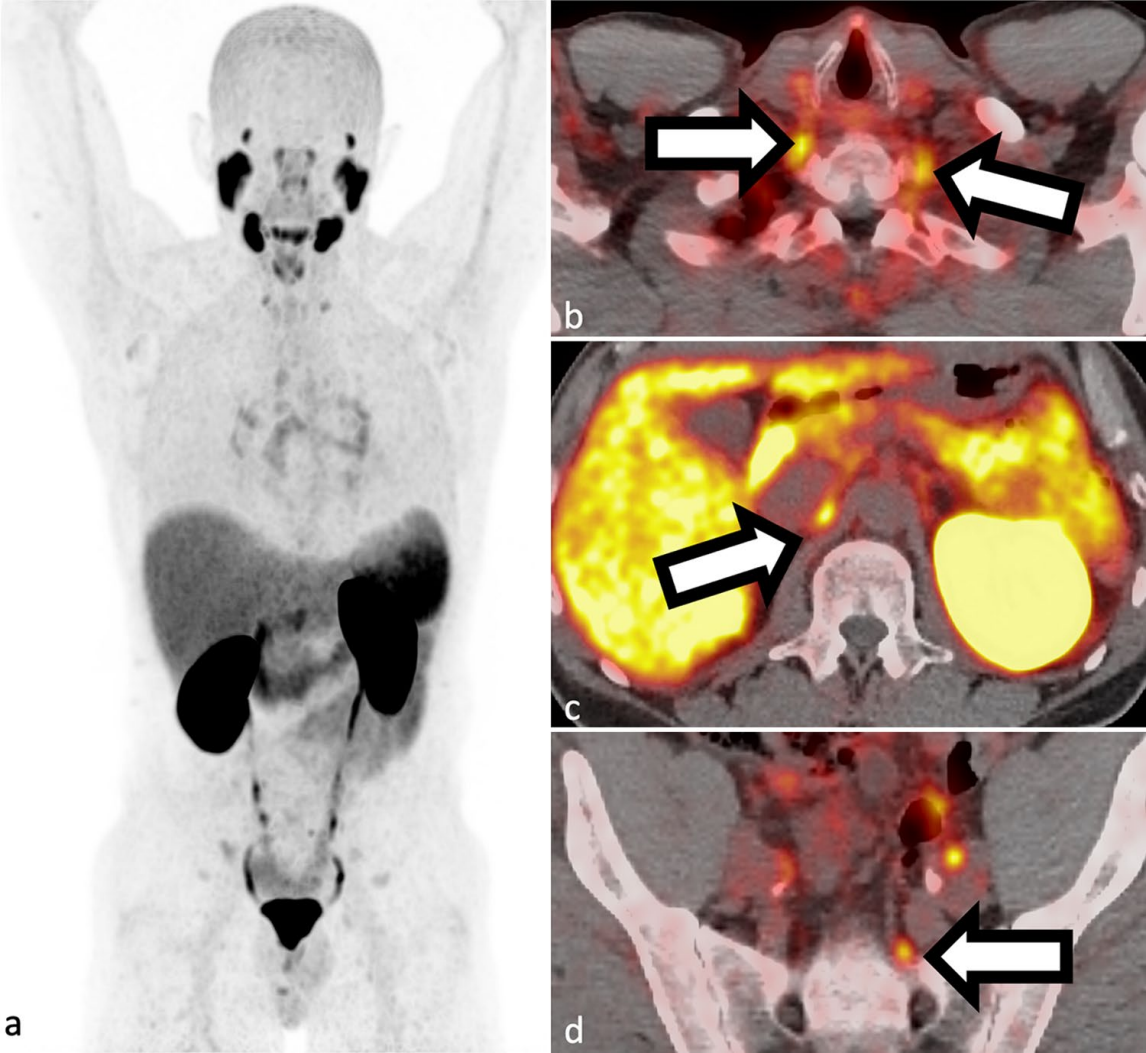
a–d Example of normal 68Ga-PSMA-11 PET biodistribution in whole-body maximum intensity projection (a SUV scaled at 0–10) with cervical (b), celiac (c) and presacral (d) ganglion uptake
